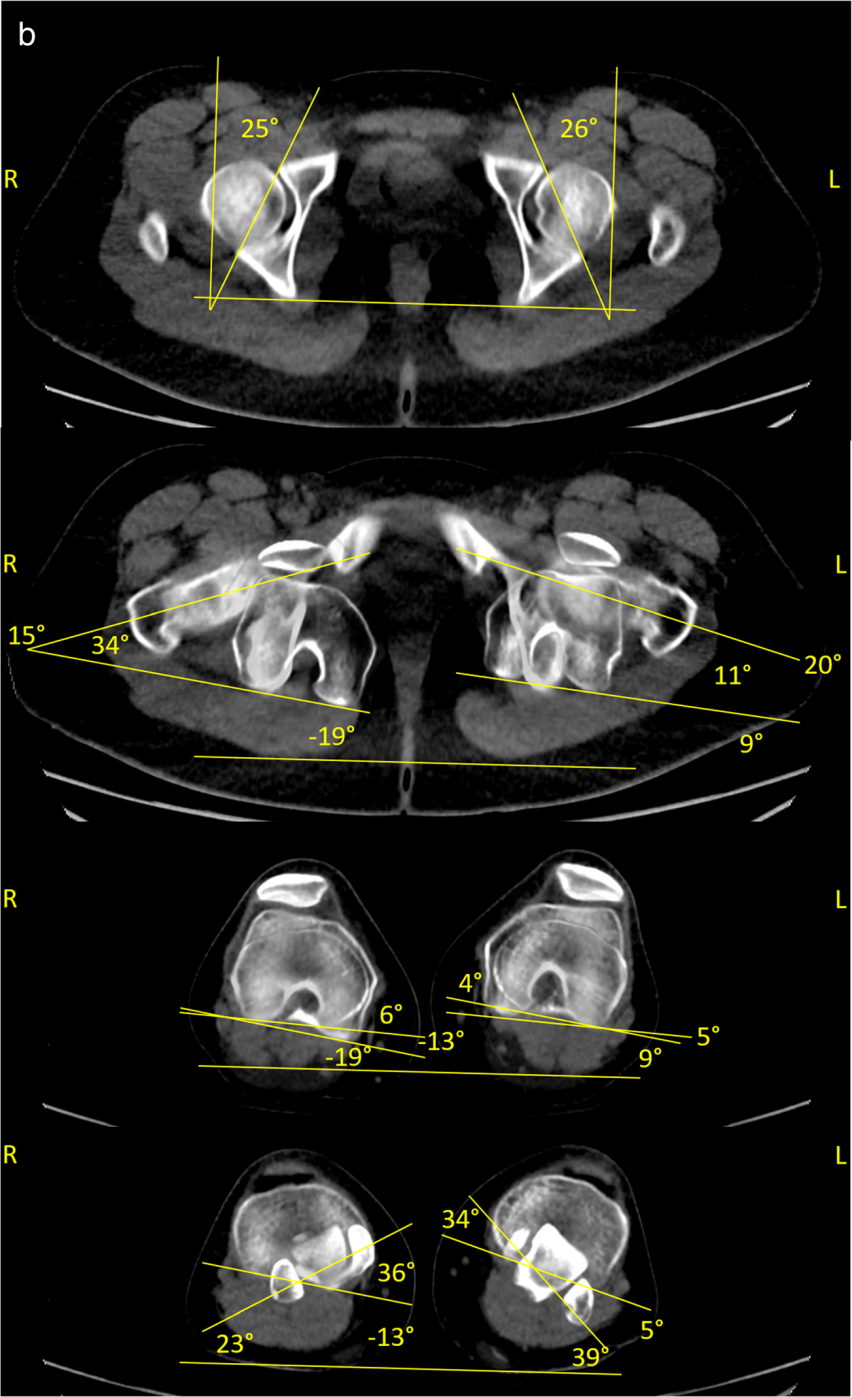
Radiographic findings in a borderline dysplastic hip patient a one of the pelvis, in anteroposterior and one axial view b rotational alignment of the lower extremity
